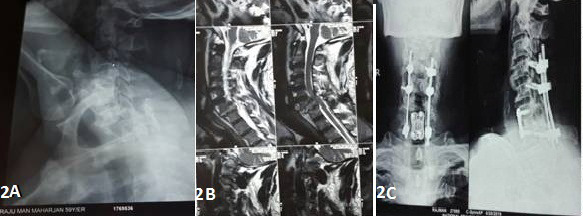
(A) Preoperative lateral x-ray of the cervical spine with fracture-dislocation of the C6-7 Level. (B) Preoperative MRI of the cervical spine with cord compression at the C6-7 level. (C) Postoperative AP and lateral x-rays of the cervical spine with anterior cervical plate, bone graft, lateral mass screws, and rod insitu.
